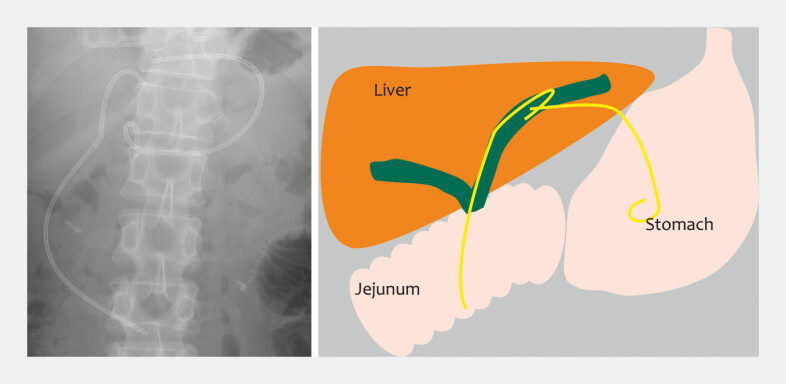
Abdominal radiography revealed that one of the stents had migrated into the biliary system.
